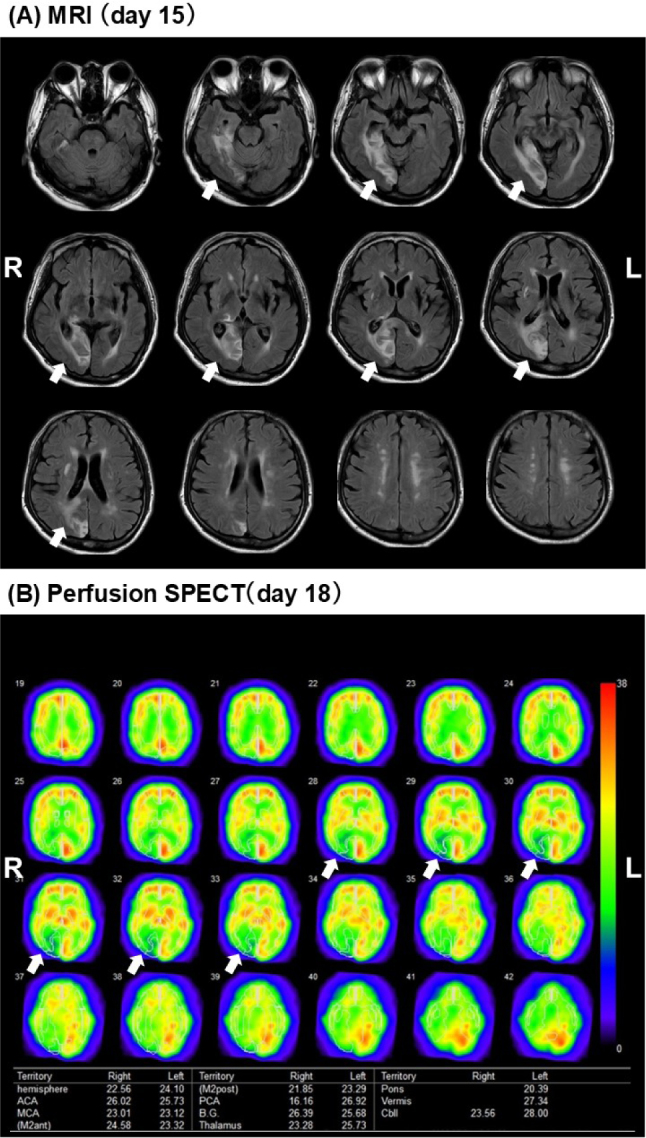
Structural magnetic resonance imaging — MRI (day 15) and perfusion single photon emission computed tomography — SPECT (day 18). MRI shows high-signal lesions in the right thalamus, medial temporal lobe, medial parietal lobe, and occipital lobe. SPECT demonstrates hypoperfusion within the right posterior cerebral artery territory and adjacent regions.
